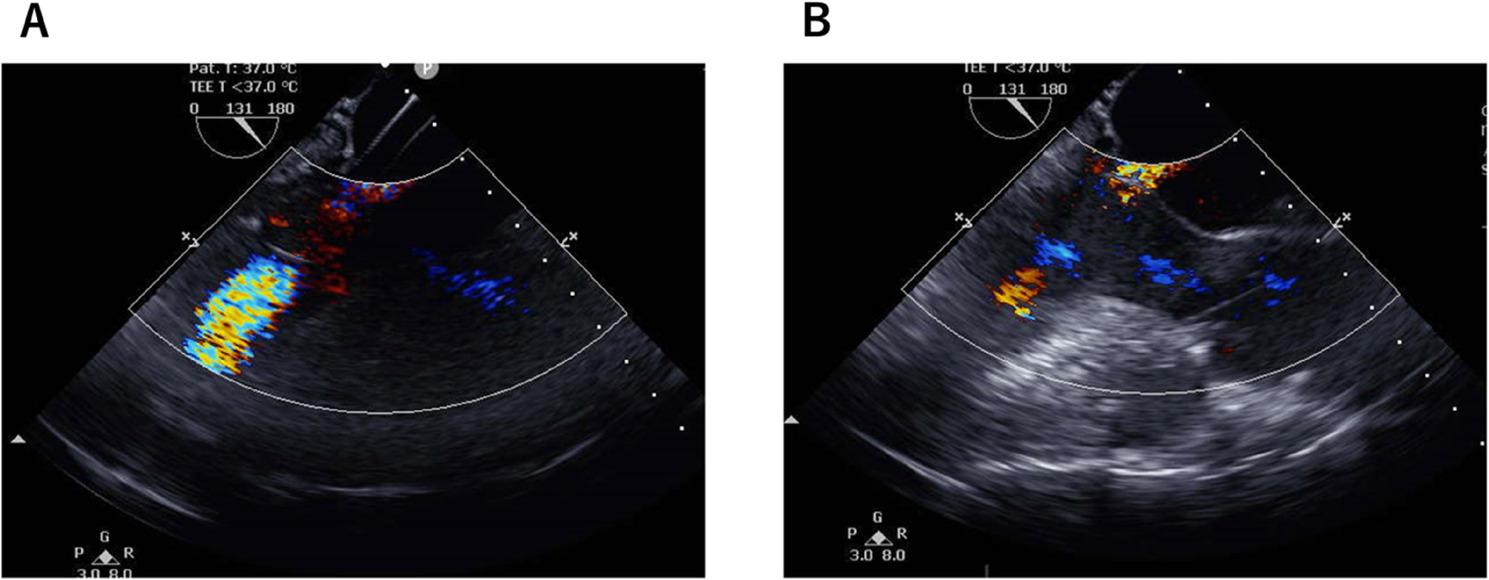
A Transoesophageal ultrasonography colour doppler imaging demonstrated a mosaic flow pattern at the tip of the arterial cannula, indicating a turbulent high-velocity perfusion jet. B It was confirmed that cannula interference did not result in severe mitral regurgitation or left ventricular outflow tract obstruction
